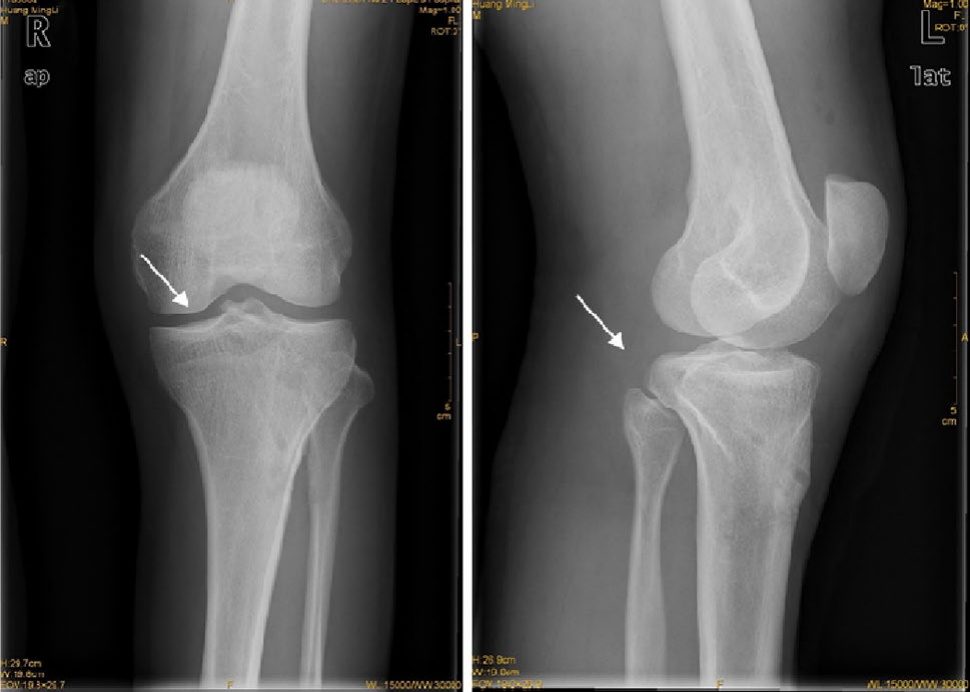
Postoperative X-ray. Postoperative X-ray shows good avulsion fracture reduction at the distal insertion of the posterior cruciate ligament (represented by the *arrow*)
